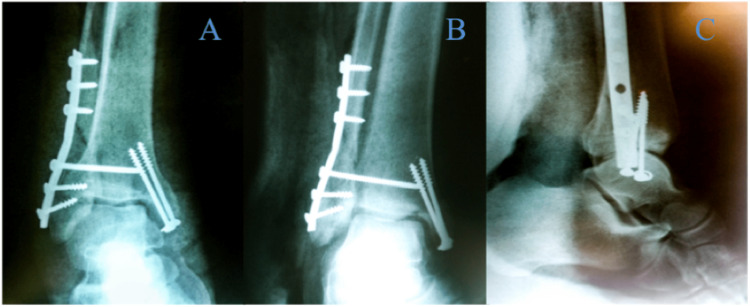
Plain radiographs of the AP, lateral and mortise views of the right ankle post-open reduction internal fixation and posterior tibialis tendon repair A - AP view, B - mortise view, C - lateral view A: anteroposterior
